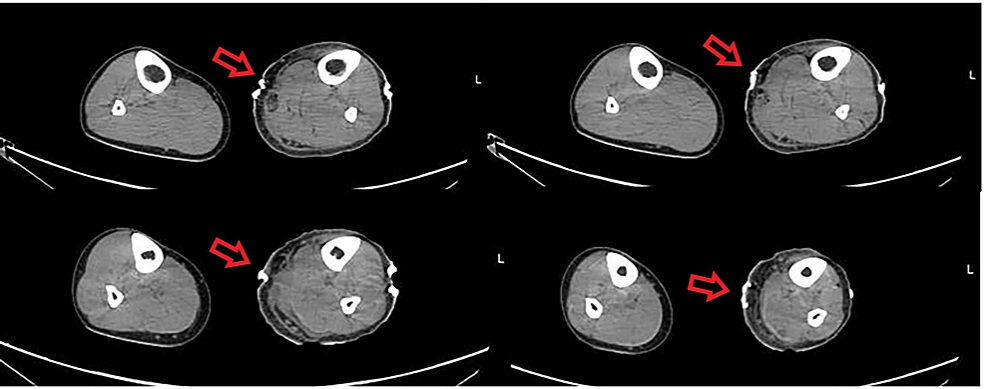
CT axial imaging: after surgery, edematous thickening of subcutaneous tissue appeared along the leg. Under the surgical metal clips, an intrafascial hydro-aerial collection is visible. CT, computed tomography
